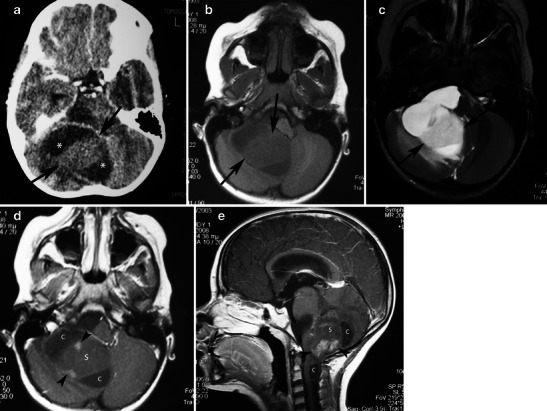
Juvenile pilocytic astrocytoma of the cerebellum in a 5-year-old girl. a Post-contrast CT shows a cystic lesion involving the right cerebellar hemisphere with a hypodense cystic component (asterisks) and a large, solid, isodense component (arrows). b On axial T1-weighted image, the solid component is homogeneous and hypointense compared with the grey matter (arrows). c On T2-weighted images, the solid component appears hyperintense compared with the grey matter and slightly hypointense compared with the cerebrospinal fluid (arrows). Post-contrast axial (d) and sagittal (e) images demonstrate heterogeneous enhancement of the solid component of the mass with areas that remain unenhanced (s) and areas with nodular enhancement (arrowheads). Note the cystic components (c)
